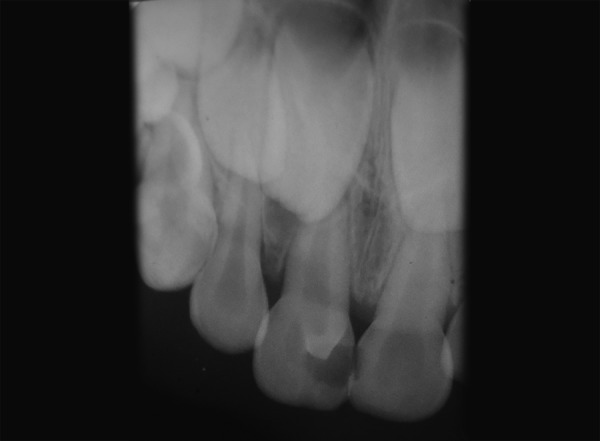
Radiographic view of talon cusp
